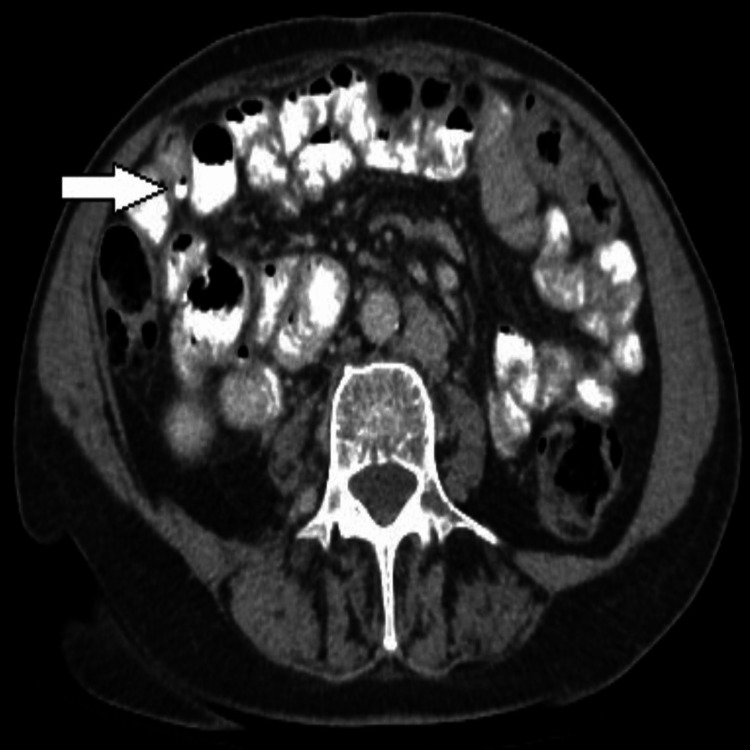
MSCT axial section (venous phase) through the lower part of the abdomen shows the diverticulum indicated by the arrowhead. MSCT: multi-slice computed tomography
